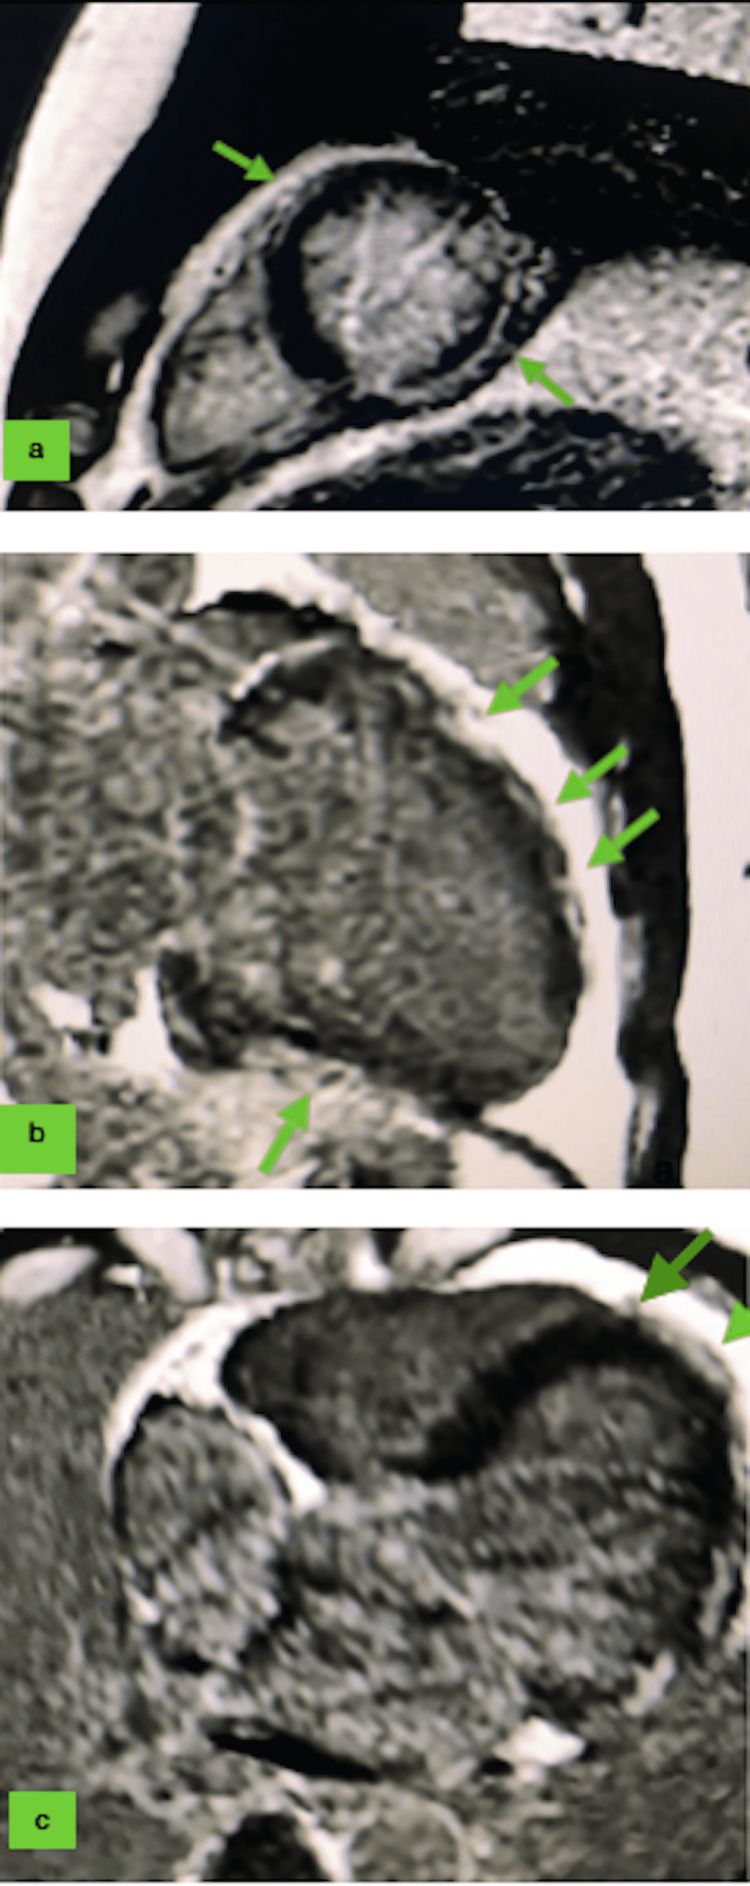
Cardiac MRI of our patient with late gadolinium enhancement showing an epicardial pattern in anterior, inferior, and lateral walls in favor of myocarditis (arrows). (a) Short axis view. (b) Two-chamber view. (c) Three-chamber view.
